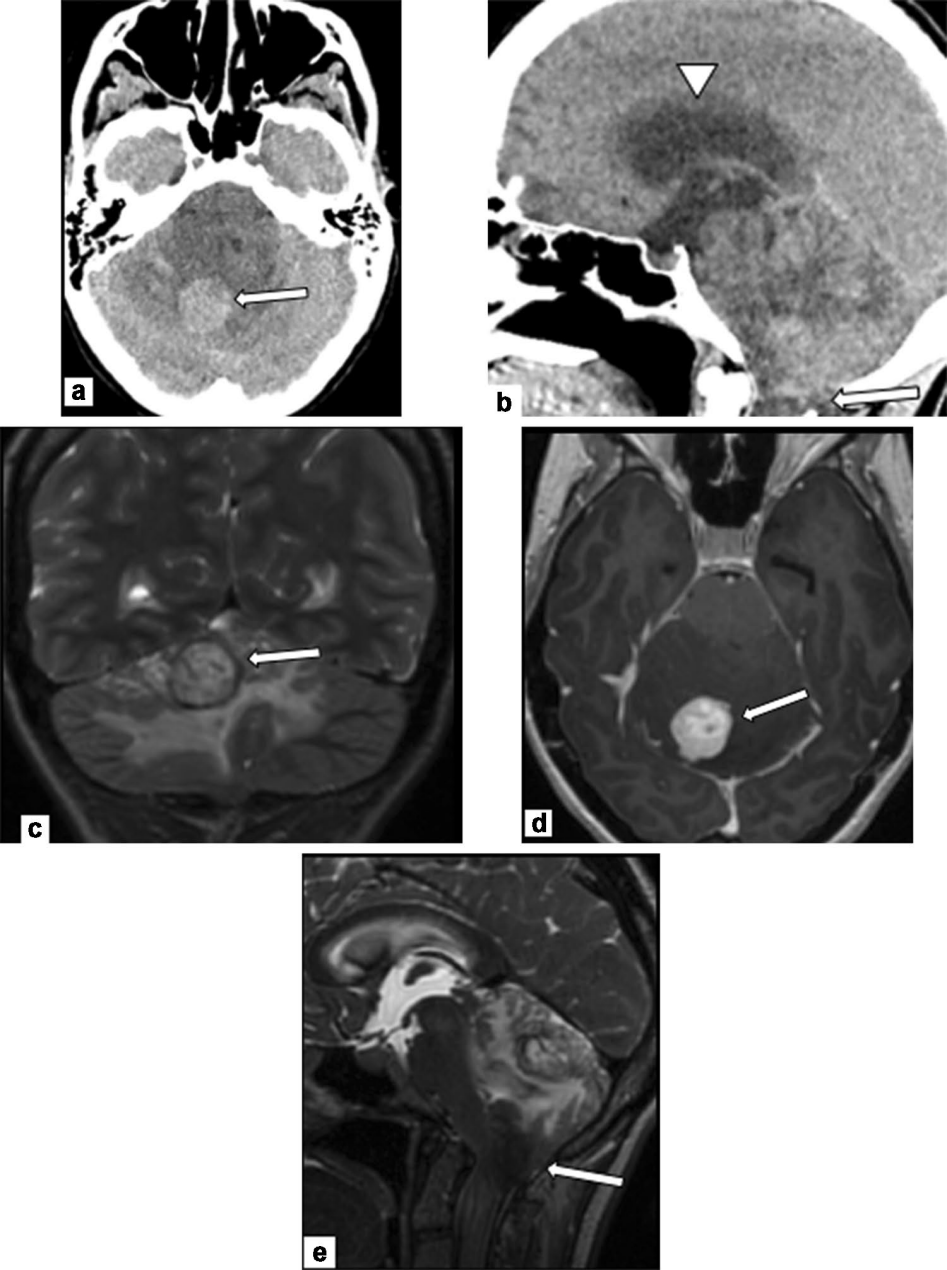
30-year-old pregnant patient, at 32 weeks of gestation, presenting with persistent headaches and nausea. a Axial non-contrast CT demonstrated a dense mass centered in the right cerebellum with surrounding edema (arrow). b Sagittal non-contrast CT demonstrated downward cerebellar tonsillar herniation (arrow) and acute obstructive hydrocephalous (arrowhead). Emergent treatment was initiated to reduce intracranial pressure. Once the patient was stable, a multidisciplinary team discussed next best steps in management. Despite theoretical fetal risks, the decision was made to pursue an MRI with contrast. c Coronal T2-weighted MRI images demonstrated a hyperintense mass (arrow) with a peripheral rim of hemosiderin and vascular flow voids. d T1-weighted contrast-enhanced MRI axial images demonstrated a well-circumscribed, enhancing mass, (arrow) most compatible with a hemangioblastoma. e Sagittal T2-weighted MRI images demonstrated continued marked mass effect with tonsillar herniation (arrow), upper cervical cord edema, and papilledema. The patient was scheduled for urgent surgery. However, the day before her surgery, she became preeclamptic and was delivered by emergency cesarean section at 33 weeks. After delivery, the patient underwent craniotomy for tumor resection with pathology confirming hemangioblastoma. After resection, the patient reported resolution of her presenting symptoms and is being followed with serial imaging
